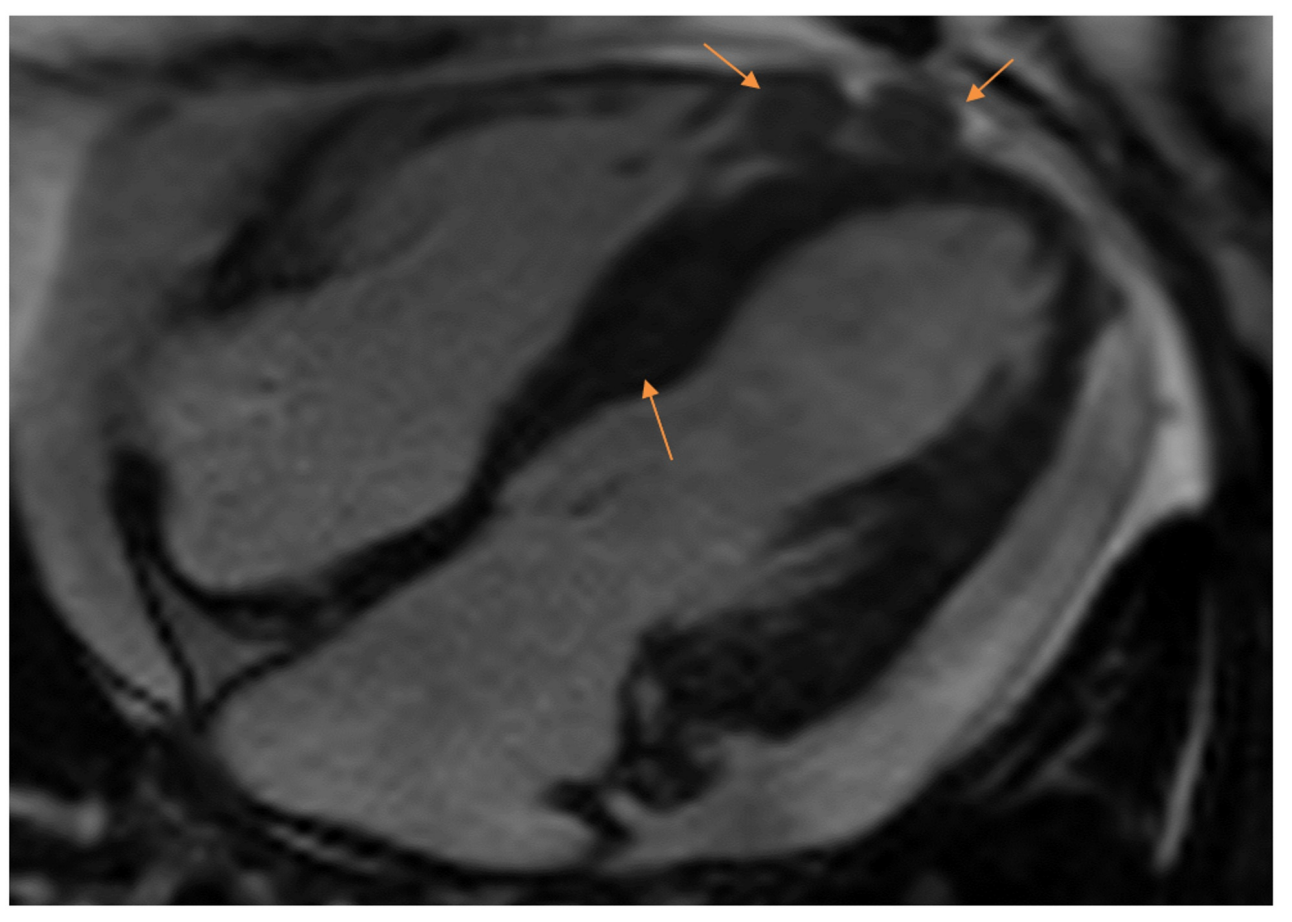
Four-chamber view of the heart as seen on cardiac MRI. Note the metastatic lesions in the right ventricular apex and the inferior interventricular septum, as indicated by the yellow arrows.
